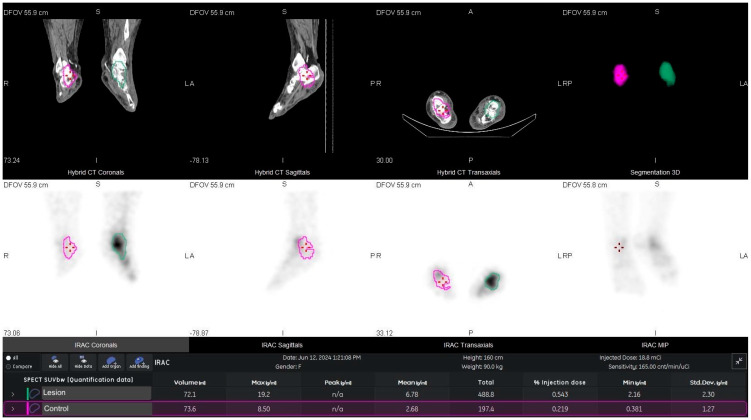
An example of SUVmax and SUVmean processing on 99mTc-MDP bone SPECT/CT with osteomyelitis on the left talus. (green line: osteomyelitis talus; purple line: contralateral talus).
